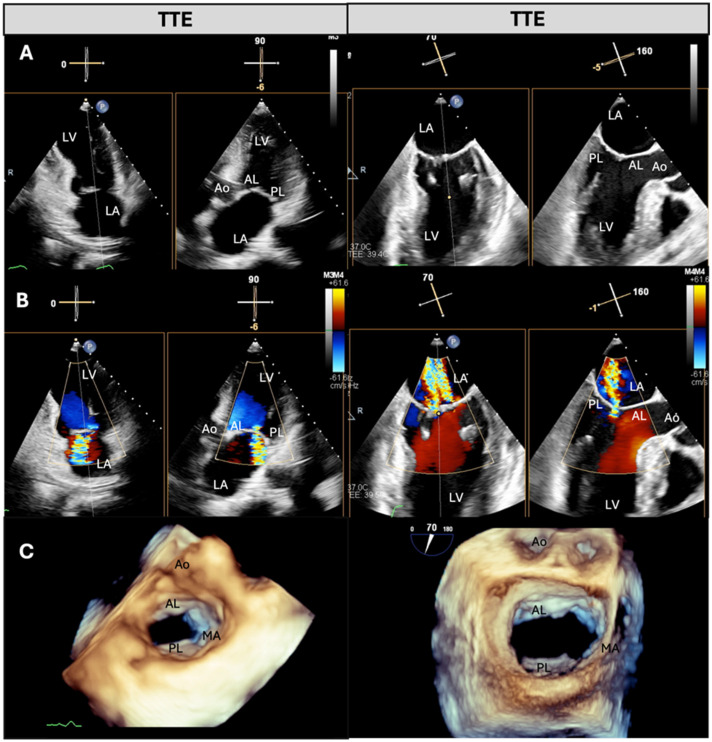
Comparison of TTE and TEE imaging in the assessment of functional mitral regurgitation. Example of functional mitral regurgitation assessed using transthoracic echocardiography (TTE, left) and transesophageal echocardiography (TEE, right). (A) B-mode images acquired using X-plane mode, showing the mitral valve structures with leaflet tethering due to left-ventricular dilation. (B) Color Doppler images were obtained with X-plane mode, showing the regurgitant jets extending into the left atrium. (C) Three-dimensional reconstructions of the mitral valve. The TEE 3D image provides higher anatomical resolution. Ao = aorta; AL = anterior leaflet; LA = left atrium; LV = left ventricle; MA = mitral annulus; PL = posterior leaflet.
